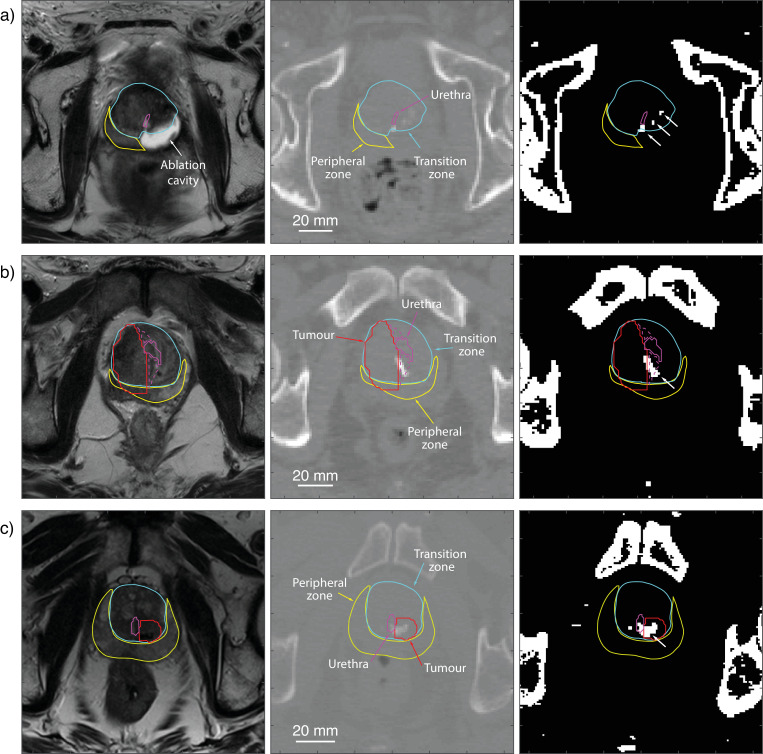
T2-weighted MR image slice (left), the corresponding CT image slice (middle), and thresholded image (right) for 3 example cases: (A) previous left-sided HIFU on which calcifications (highlighted by white arrows) are visible within the transition zone midgland contour (blue). (B) Calcification is visible between the urethra and tumor. (C) Calcification is present within the tumor. The peripheral zone midgland (blue), urethra (purple), tumor (red), and area between urethra and tumor (purple dotted) are also shown. (Color version of figure is available online.)
